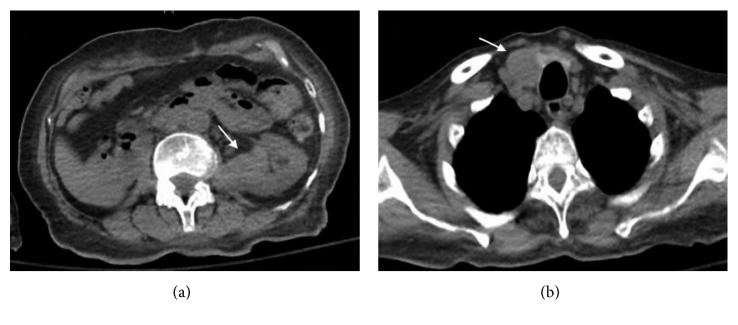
(a) Axial CT scan of the abdomen showing a mass lesion involving the inferior pole of the left kidney (arrow), breaching the renal capsule and infiltrating into adjacent retroperitoneal space. (b) Axial CT scan of the chest at the level of thyroid showing a well-defined hypodense nodule in the right lobe of the thyroid gland (arrow).
